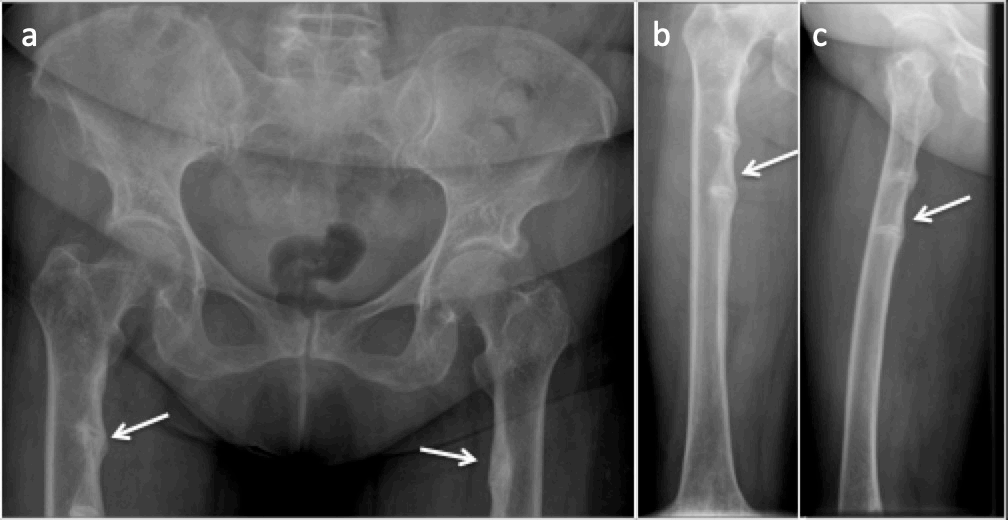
(a) Anteroposterior radiograph of the pelvis. (b) Anteroposterior and (c) Lateral radiograph of the right femur showing looser zones of osteomalacia. Multiple, bilateral lucency which involve only a part of femoral shaft along the medial aspect, oriented perpendicular to the cortex with associated surrounding sclerosis.
